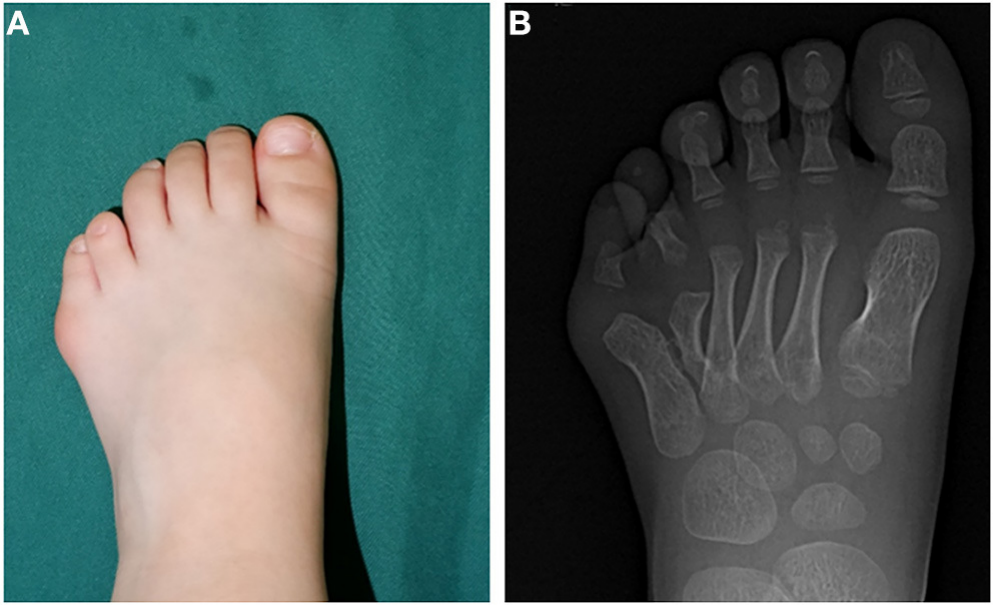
Preoperative morphological and imaging features. (A) Preoperative photos depicting the appearance of the affected foot. (B) Preoperative radiograph showing malformed fifth metatarsal and wide forefoot.
